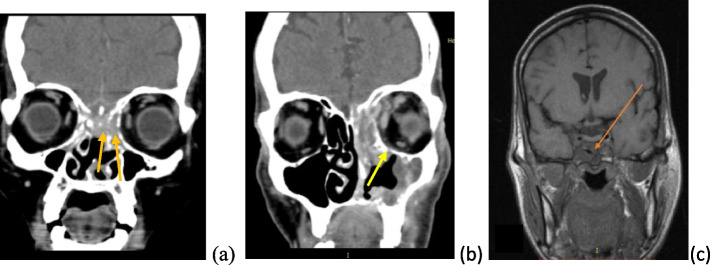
a–c Radiological investigations of prototype cases of case series 4. a CT PNS shows hypodense opacities in bilateral ethmoids with erosion of cribriform plate bilaterally. b CT PNS of a patient with rhino orbital mucormycosis showing (L) intra orbital abscess with defect in cribriform plate. c MRI (T1 weighted) image of a patient with rhino orbital cerebral mucormycosis with cavernous sinus thrombosis (R)
